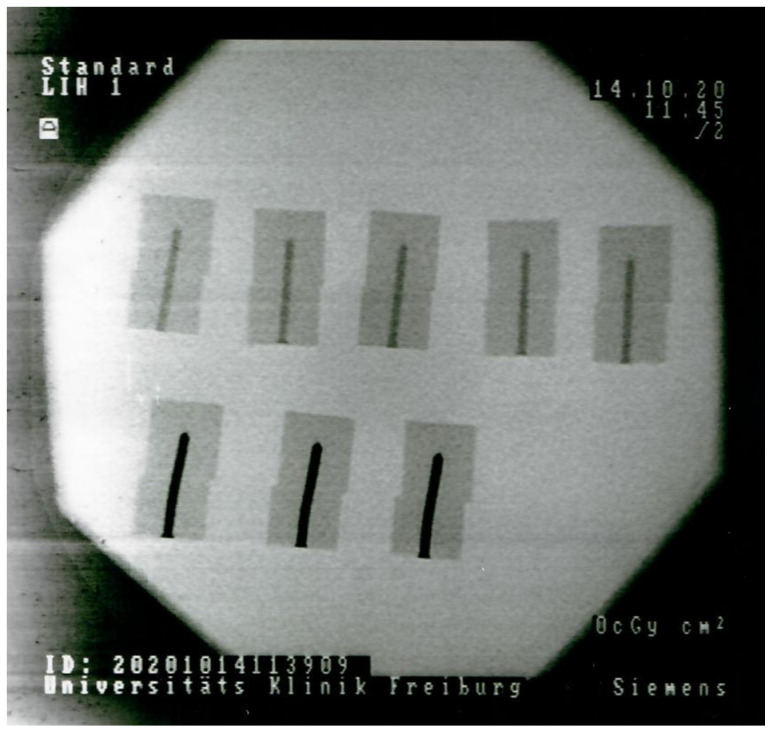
X-ray of the zinc pins (down low) and the MAGNEZIX pins (up high) after quasi-static testing and failure with Siremobil-ISO C from Siemens (Siemens AG, Munich, Germany).
